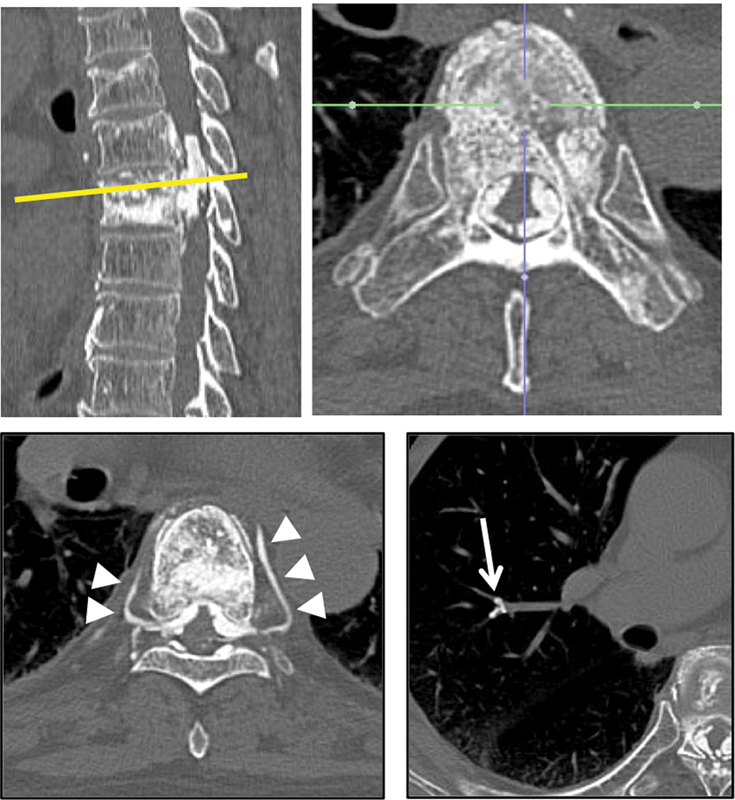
Upper left panel: preoperative computed tomography (CT) sagittal image showing leaked polymethyl methacrylate (PMMA) into spinal canal from T6 thorough T7. Upper right panel: preoperative CT axial image showing circumferentially leaked PMMA surrounding the thecal sac. Lower left panel: axial CT image showing leaked PMMA into the segmental vein bilaterally (white arrow head). Lower right panel: axial CT image showing leaked PMMA into right pulmonary artery (white arrow).
